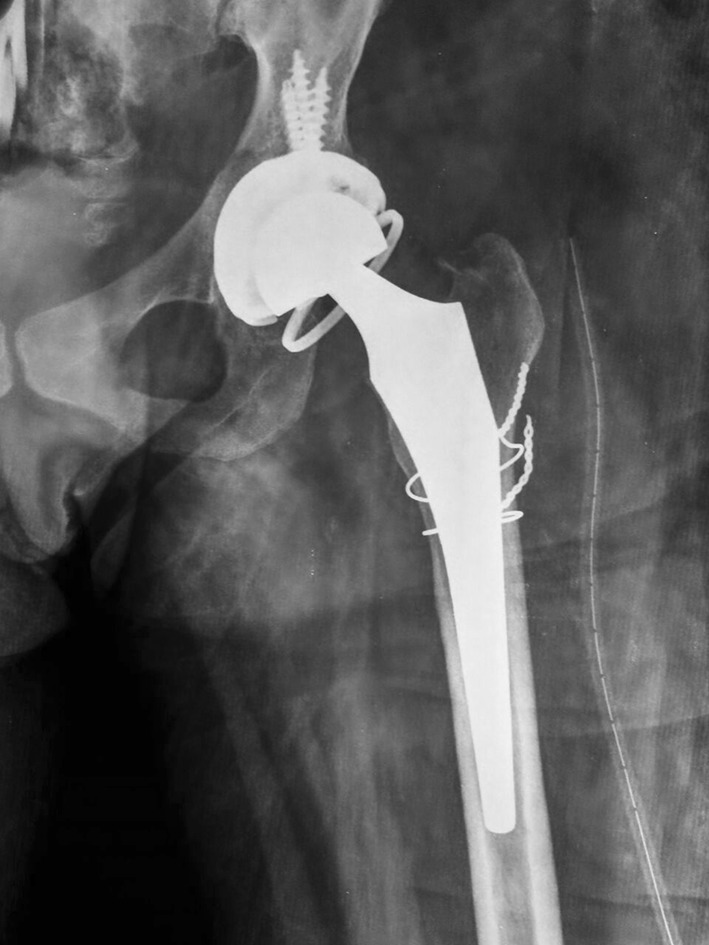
Postoperative X-ray of the patient with total hip replacement with constrained acetabular component
